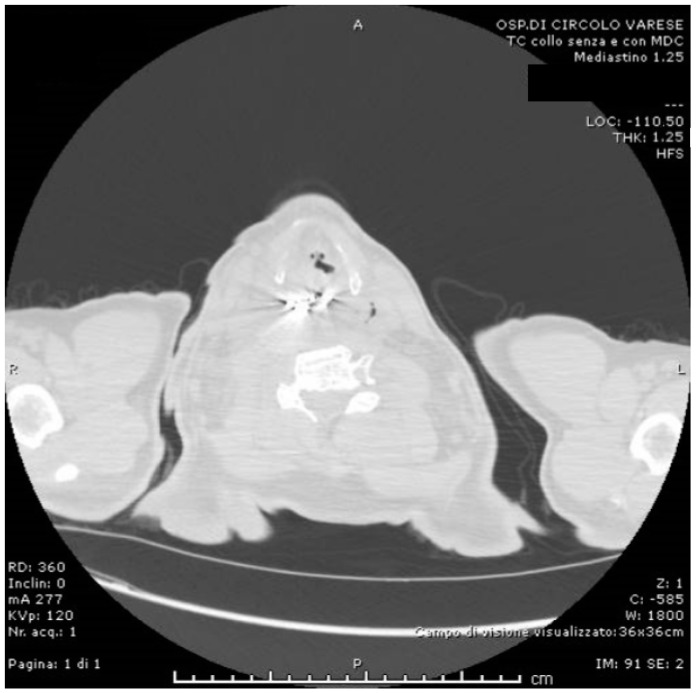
Preoperative CT scan (sagittal, lung and air view-window).
